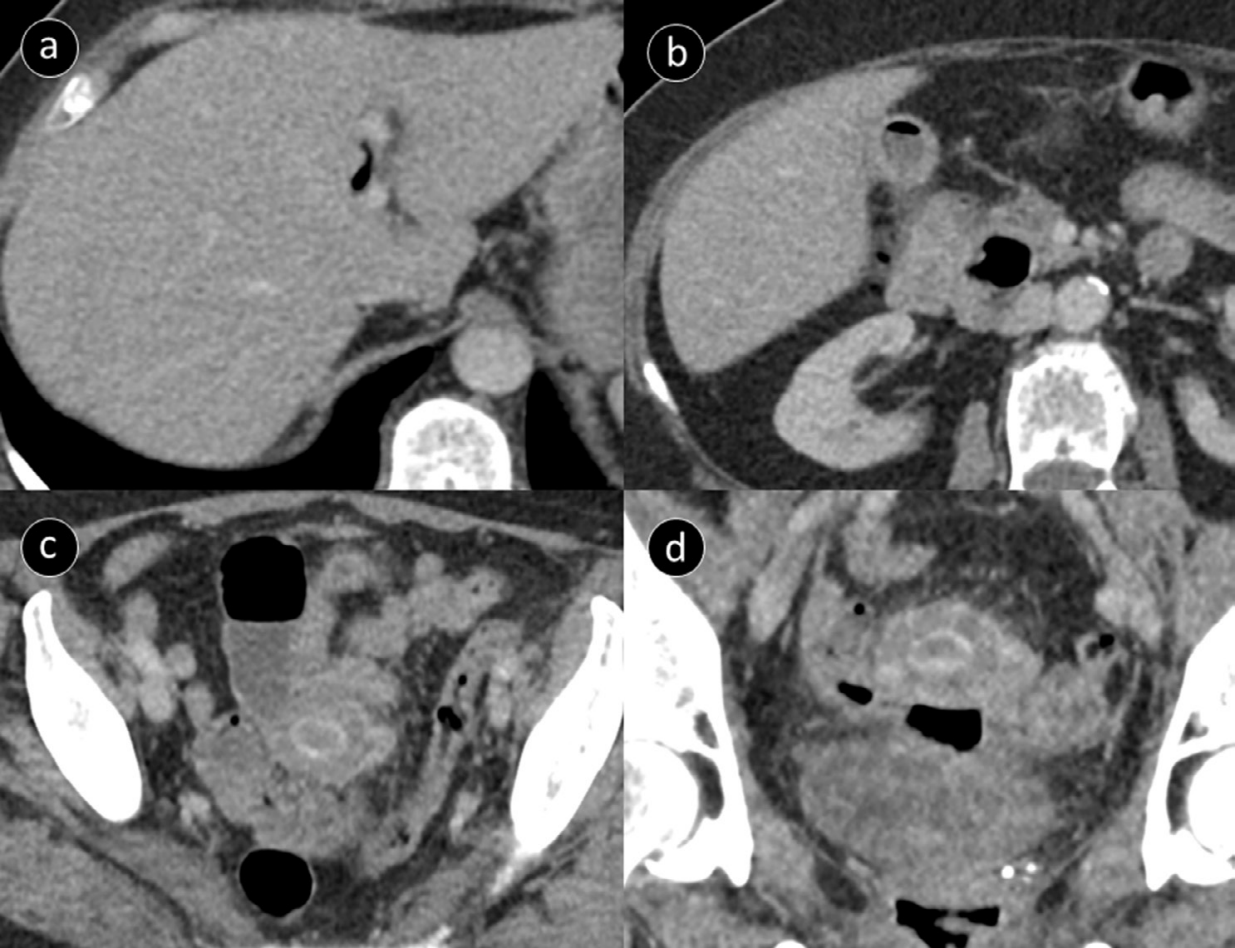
Contrast-enhanced CT performed shortly after admission. (a, b) axial image showing small volume air within the intrahepatic biliary tree and the now empty gallbladder containing air. (c, d) axial and coronal images of the laminated gallstone within a dilated loop of small bowel, note the proximity to the vagina.
